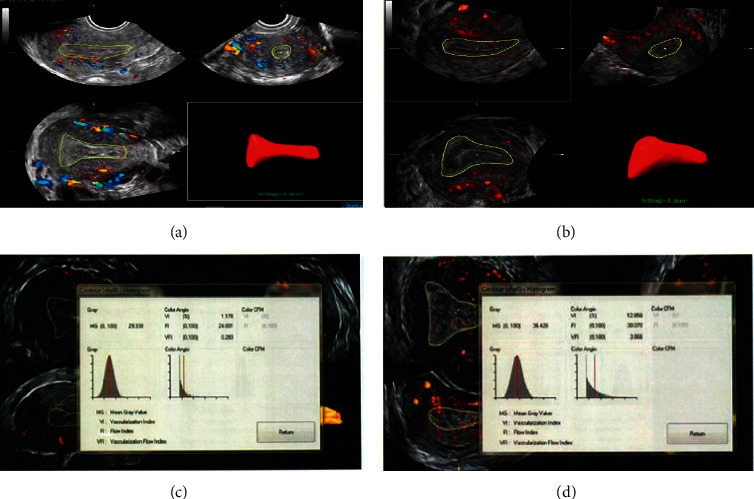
(a, b) After three-dimensional power Doppler imaging, the endometrial volume of the two groups was measured by voice software. (c) (d) After three-dimensional power Doppler imaging, the blood flow histogram function was used to obtain the endometrial VL, FI, and VFI of the two groups.
